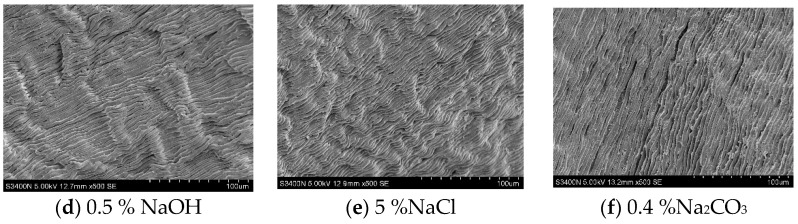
Effect of pretreatment methods on microstructure of wolfberry by ultrasonic far-infrared synergistic drying.
